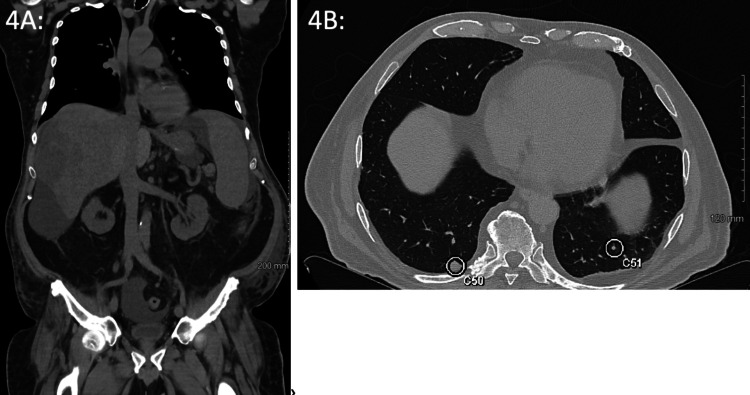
CT of the Chest, Abdomen, and Pelvis Without Contrast After TACE. *4A: *Cirrhosis of the liver with ascites and splenomegaly is present. Hypoenhancing mass is again seen in the posterior right lobe of the liver involving segments 8, 7, 6, and 5. The mass infiltrates liver parenchyma and is difficult to measure, but is larger in this study with a portion of the liver in the subdiaphragmatic region measuring 133 mm and previously measured 116 mm. Previous exams also showed a tumor thrombus in the right hepatic vein, but this cannot be seen in this study. The gallbladder and biliary system, pancreas, adrenal glands, kidneys, and retroperitoneum are normal, except for the borderline enlarged portacaval node, which measured 9 mm on the previous exam and now measures 10 mm. There is a definite lytic bone lesion in the midline of the S1 sacral segment showing no change from the prior exam, consistent with bone metastasis.* 4B:* This exam demonstrates 56 pulmonary nodules with previous initial CT imaging only showing four nodules. There is an interval increase in the pre-existing nodules. A pleural-based nodule on the posterior sulcus of the right lower lobe measures 11 mm in diameter and previously measured 6 mm (C50). Another pulmonary nodule is labeled as C51.
